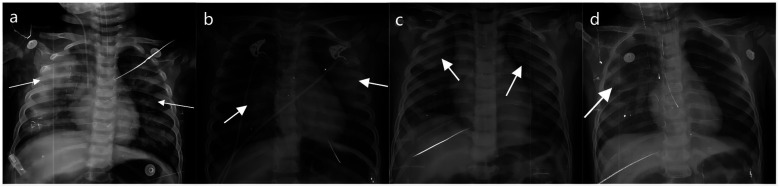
The chest radiograph of NPE in fatal cases of HFMD. (a–d) Shows different degrees of NPE in children with HFMD. The arrow points to the lesion.
